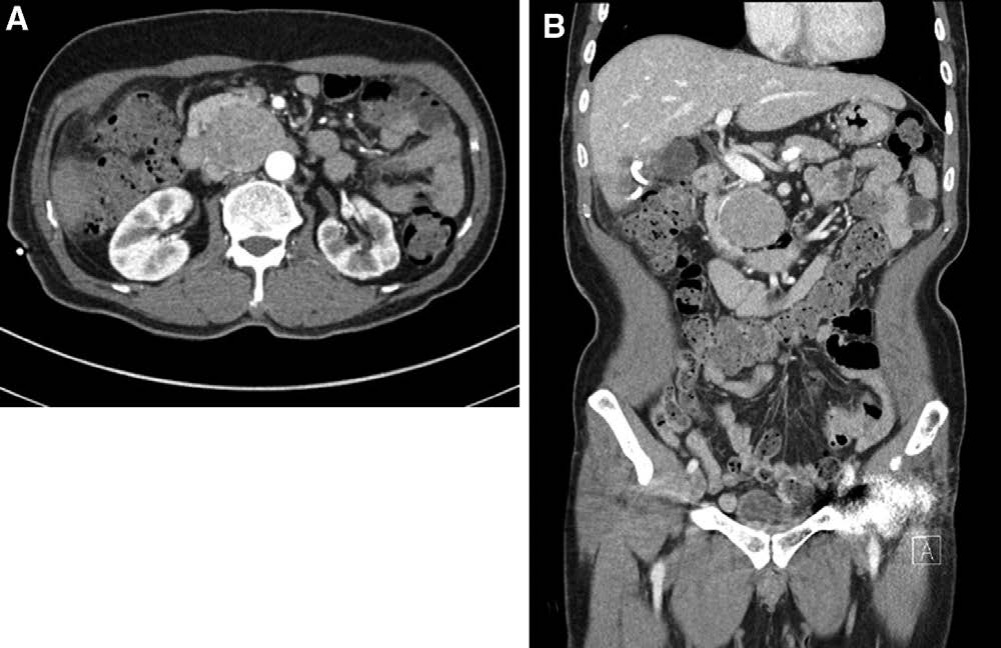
Abdominal CT taken before hospitalization. A solid mass of approximately 5 cm in size is observed in the aortocaval area. (1A) Axial plane and (1B) coronal plane.
